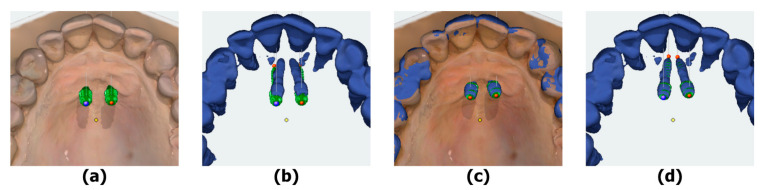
Postoperative superimposition and measurements (e.g., blue mark = “Point 1”): (a) presurgical planning model with digital OMIs; (b) digital OMIs and postoperative CBCT dataset; (c) postsurgical superimposition; (d) digital OMIs matched with the postsurgical CBCT dataset.
